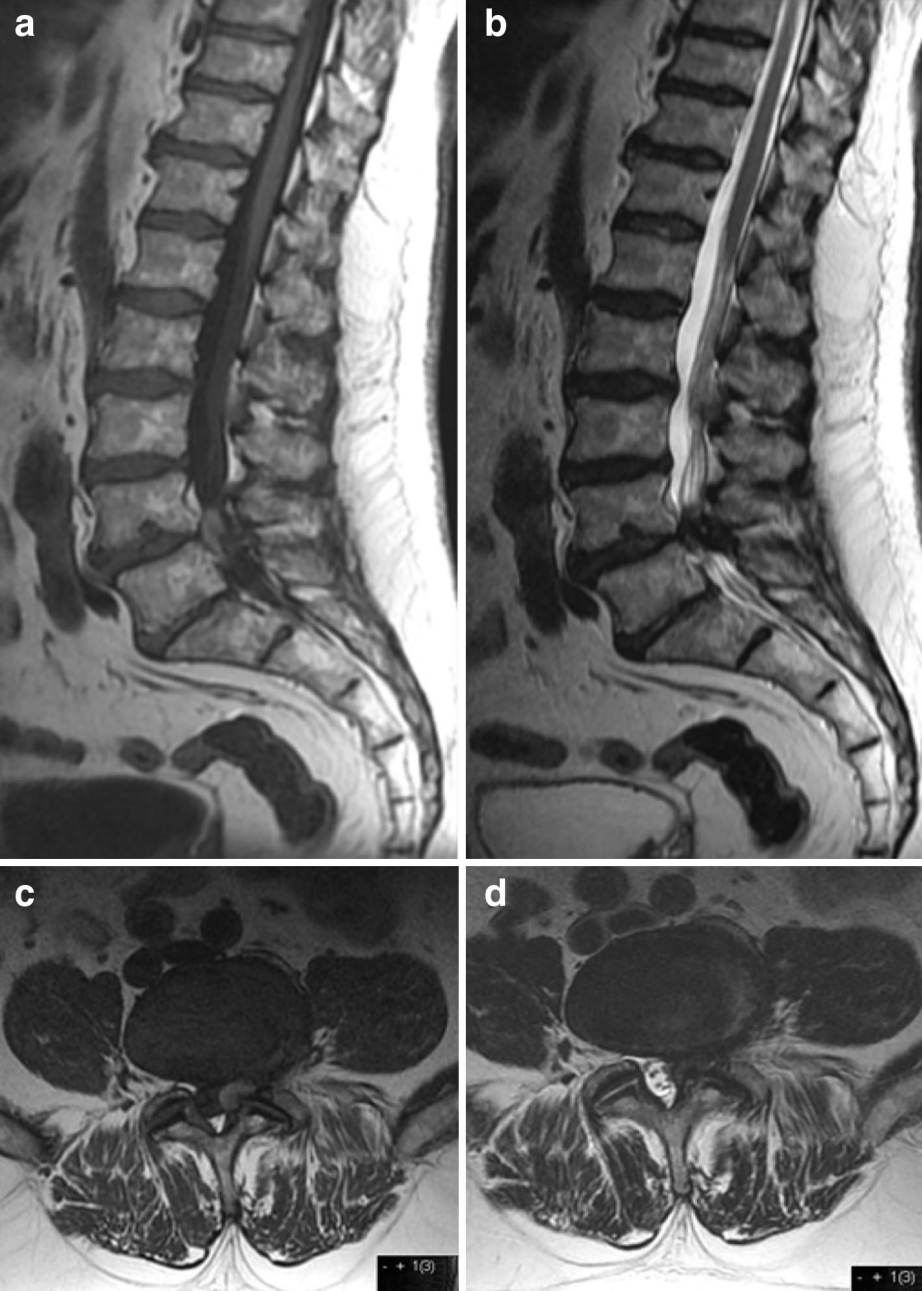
Sagital T1-weighted (a) and T2-weighted (b) axial T1-weighted image (c) and axial T2-weighted image (d) demonstrating a high signal intense epidural hematoma on the left side lumbar level L4–L5 and compressing the left L5-root
